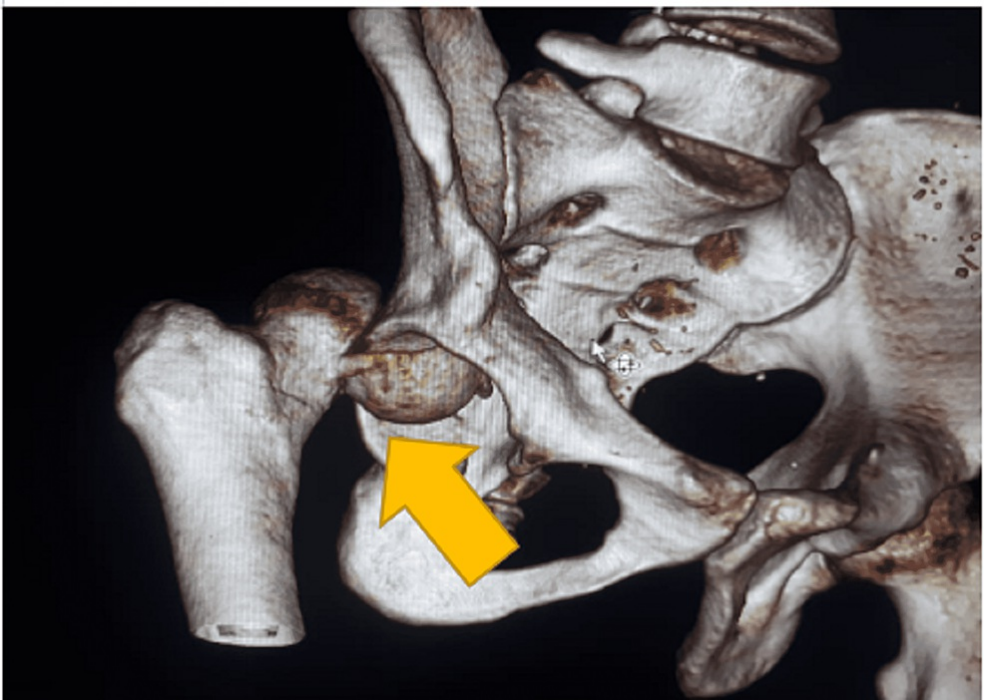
Three-dimensional reconstruction CT image showing posterior dislocation with Pipkin’s type II fracture.
